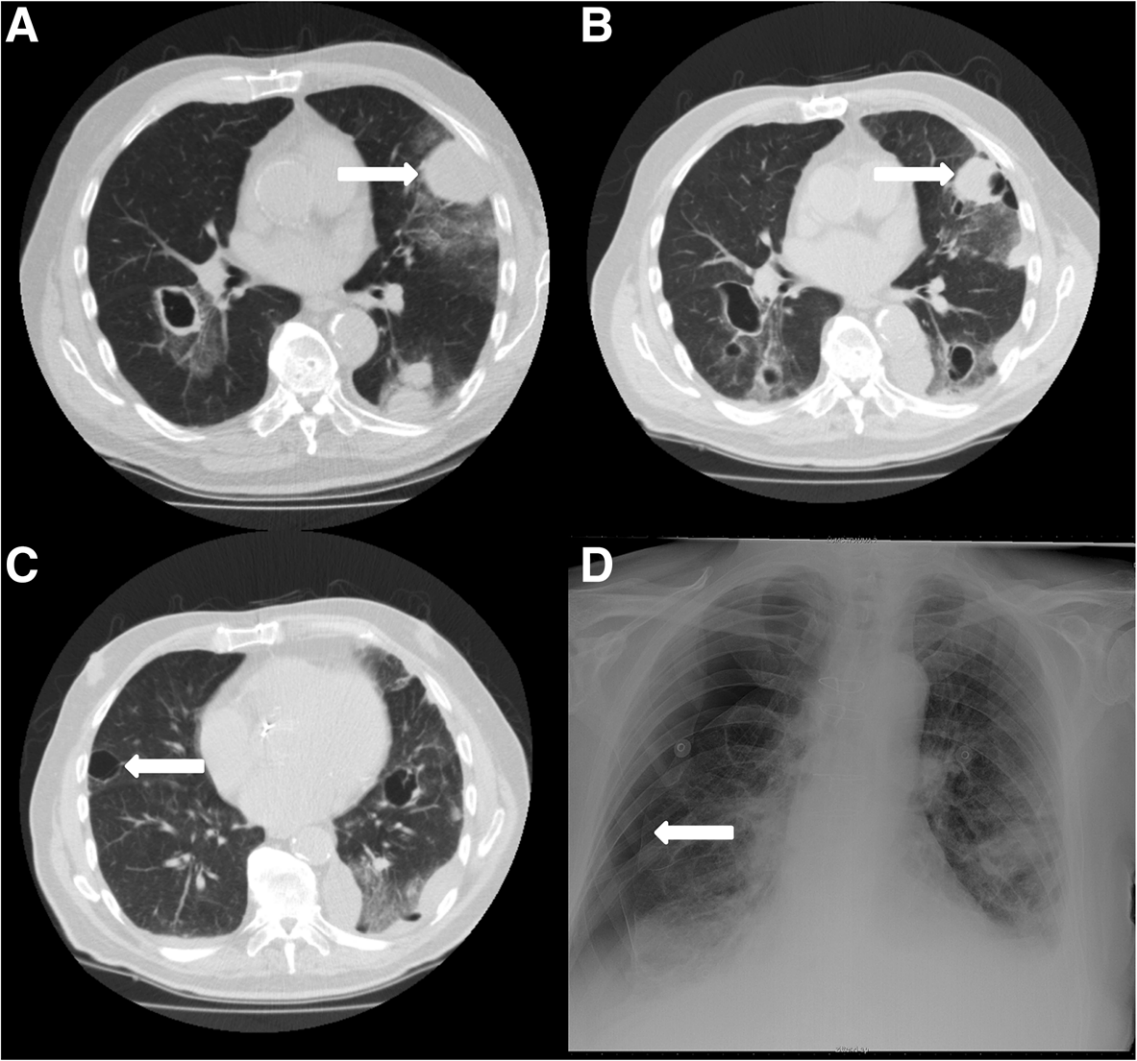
CT scans and X-thorax of patient 2. A: CT scan at start of pazopanib showing metastases in both lungs with a large necrotizing metastasis on the left side. The pleural metastasis indicated by the arrow is also visible in B, which is a CT scan after 3 months of pazopanib treatment, now showing cavitations. C is also an image from the CT scan after 3 months of pazopanib treatment showing a metastasis (arrow) with cavitation next to the pleura. D: The X-thorax shows the right sided pneumothorax. The visceral pleural line is indicated by the arrow.
